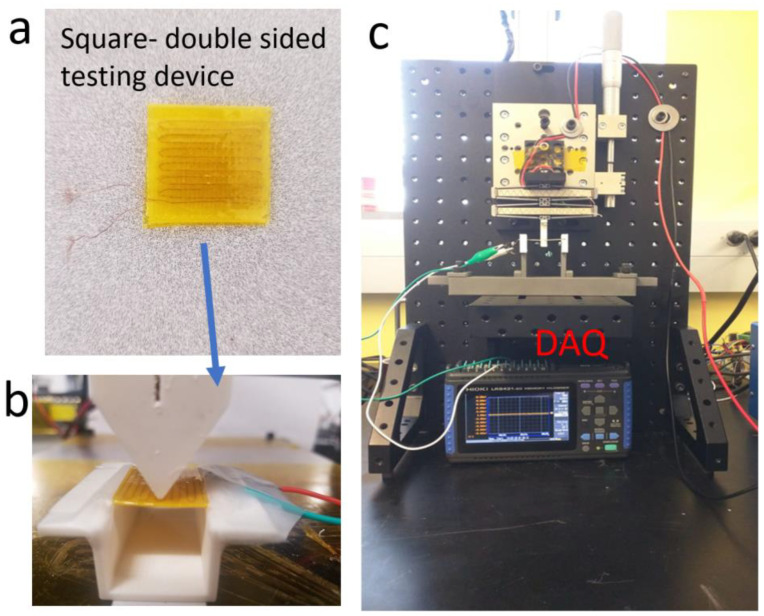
(a) Square-shape double-sided testing device with PVDF and ultrasound embedded copper wires. (b) Tooling setup. (c) Testing apparatus with vibration control and data acquisition device.
